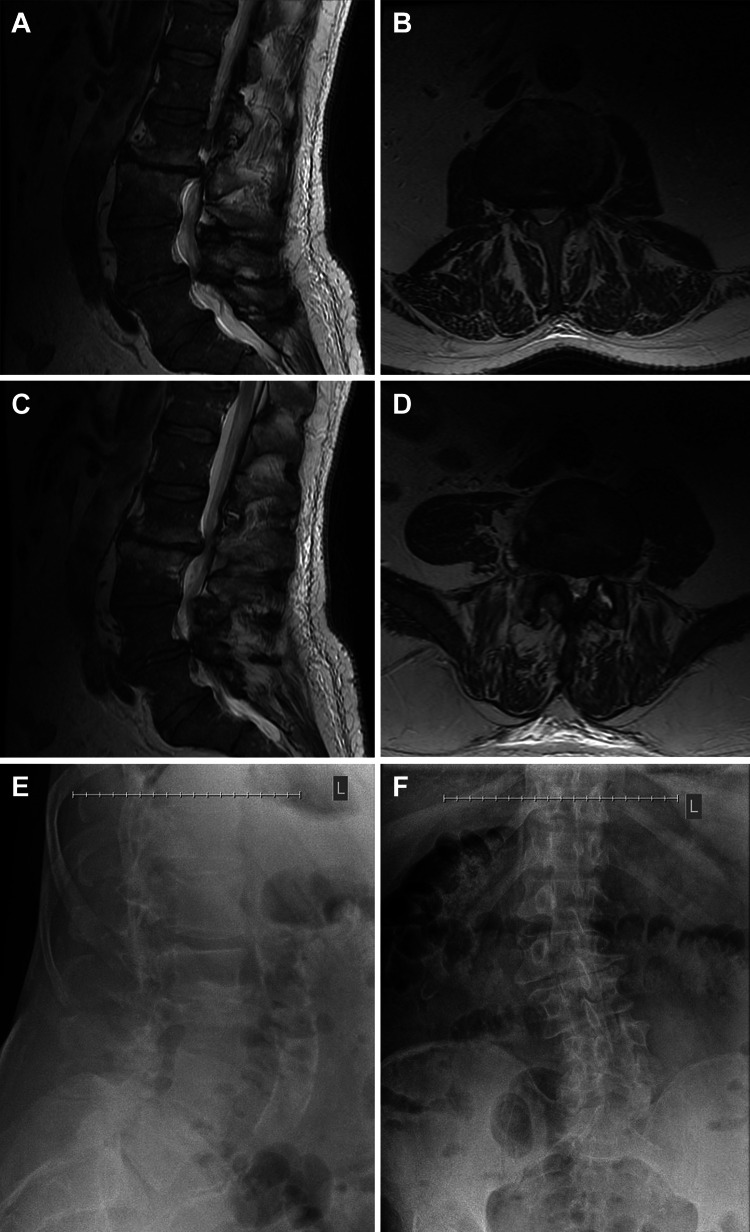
Preoperative lumbar imaging of the patient. Magnetic resonance images showing severe central and foraminal stenosis at L2-3 on (A) sagittal and (B) axial views, and at L4-5 on (C) sagittal and (D) axial views. (E) Lateral and (F) anteroposterior radiographs demonstrating dextroscoliosis, grade 1 L2-3 retrolisthesis, and grade 1 L4-5 anterolisthesis. The image is used with permission from Barrow Neurological Institute, Phoenix, Arizona.
